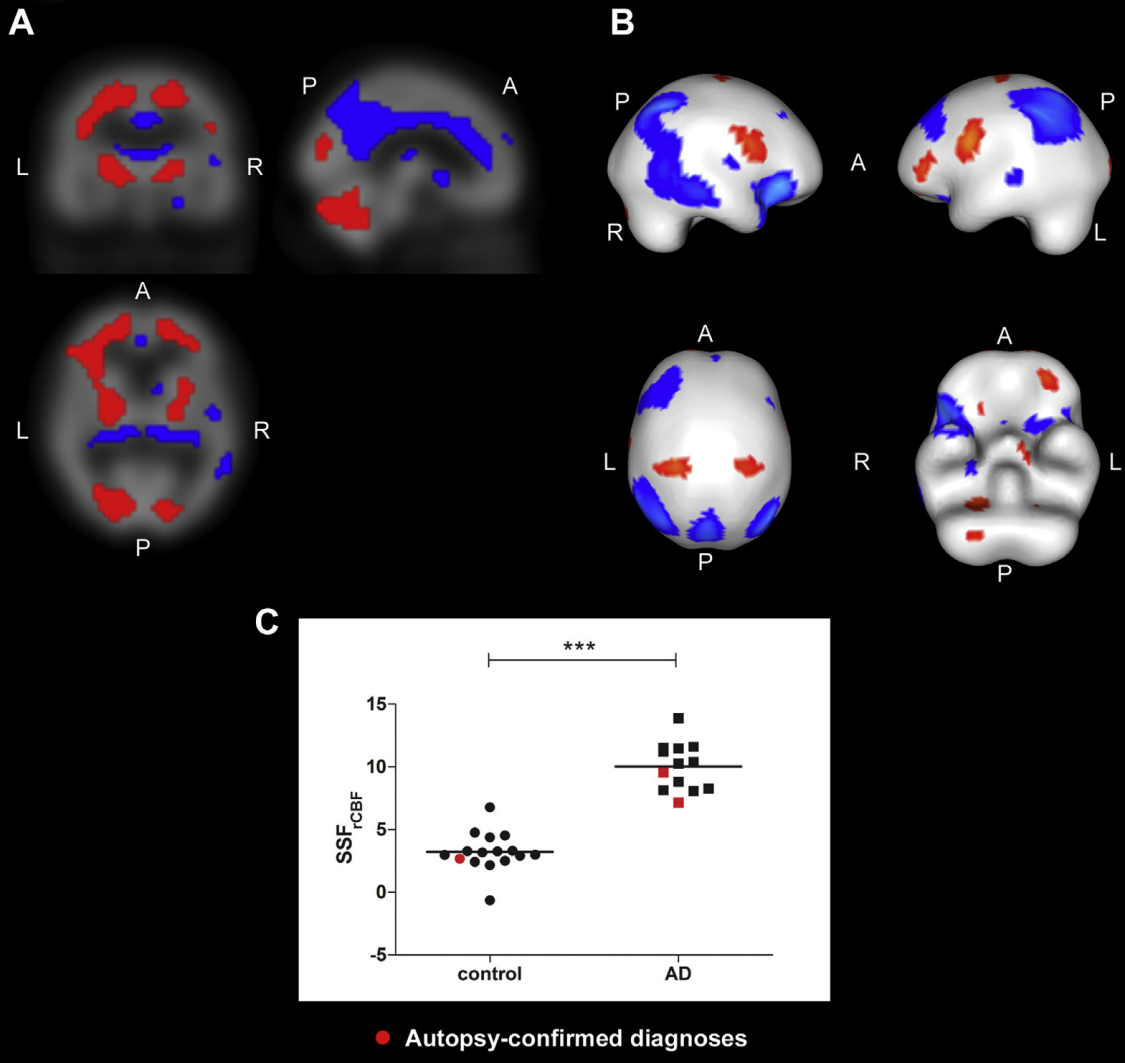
Regional cerebral blood flow spatial covariance pattern in AD (|Z| ≥ 1.64, *p* ≤ 0.05). Orthogonal (A) and rendered (B) views of the rCBF spatial covariance pattern superimposed upon the ^99m^Tc-exametazime SPECT template distinguishing AD from controls. Red and blue regions depict relative increased and decreased activity respectively. (C) Distribution of SSF_rCBF_ scores in controls (n = 16) and AD (n = 13) (p* < 0.001). Abbreviations: A, anterior; AD, Alzheimer's disease; L, left; P, posterior; R, right; rCBF, regional cerebral blood flow; SSF, subject scaling factor. (For interpretation of the references to color in this figure legend, the reader is referred to the Web version of this article.)
